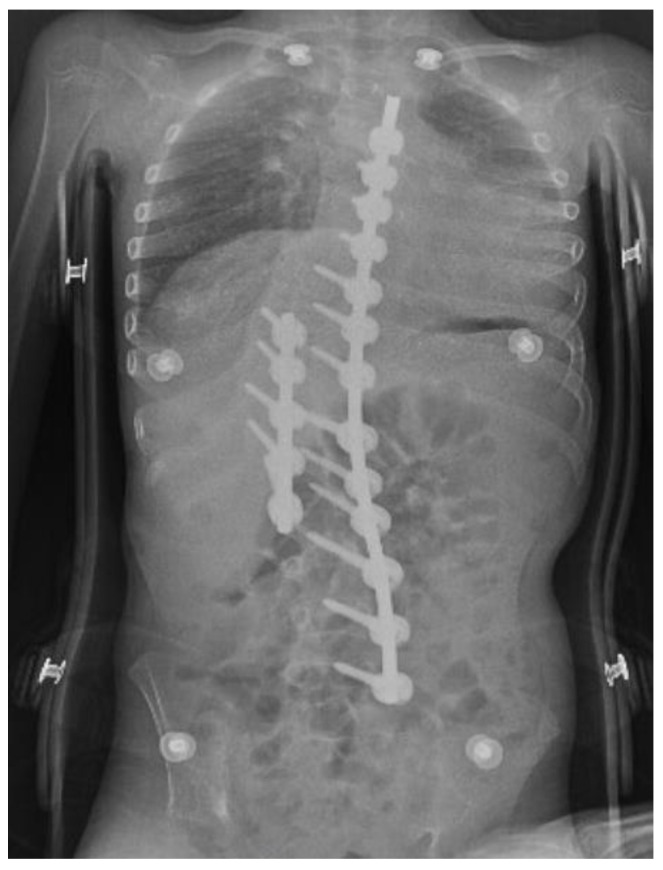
X-ray after surgery with instrumentation in the correct position.
